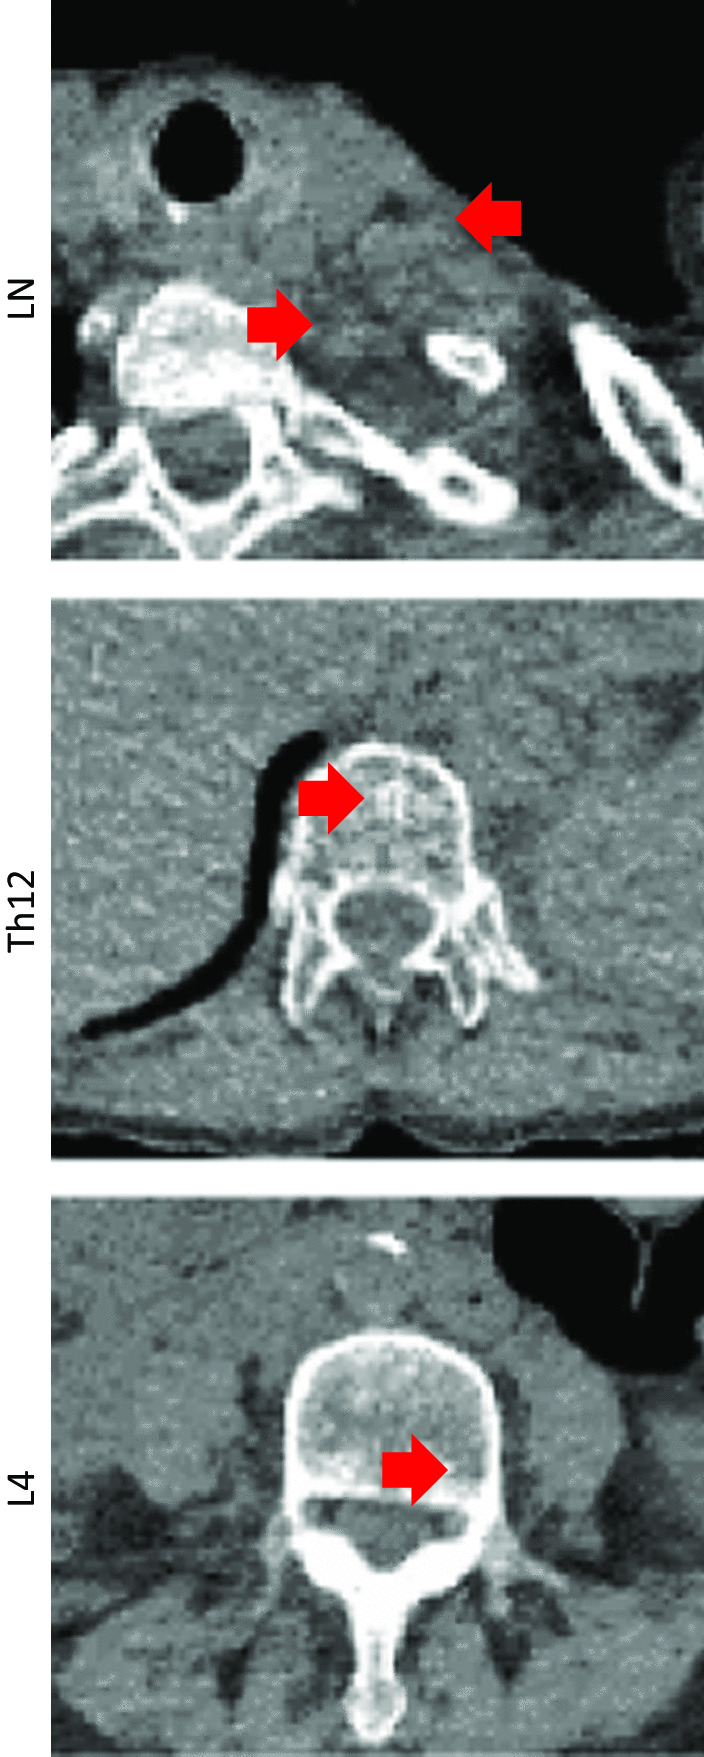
Native CT images before irradiation. The periclavicular lymph node (LN) metastases and the bone metastases in vertebrae Th12 and L4 (red arrows) are shown in axial plane
